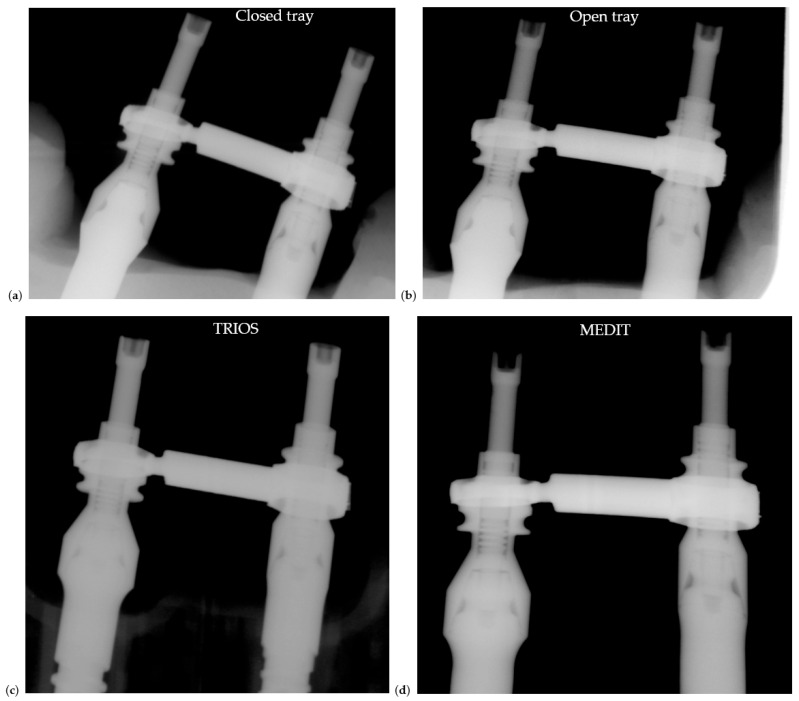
Radiographic comparison of soldered test bars on models fabricated with different impression techniques: (a) closed tray conventional impression, (b) open tray impression, (c) TRIOS scan and (d) MEDIT scan.
